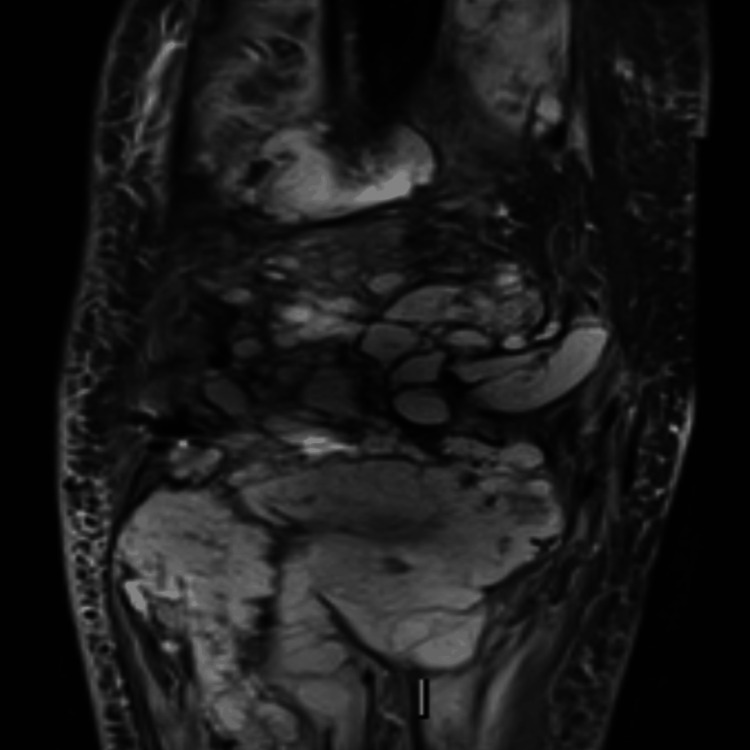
Coronal T1 fat-suppressed MRI of the right knee. The image demonstrates a large soft tissue mass involving the bones and soft tissue of the right knee. Artefacts from the prosthesis can be noted.
